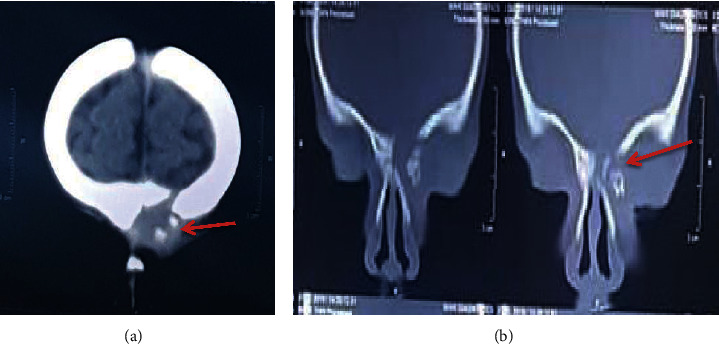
CT scan head of the infant. (a) Defect in the frontal bone; there is discontinuity of the left frontal bone with erosions. (b) Erosion of the frontal bone plate due to chronic postinfective etiology as seen on CT head, suggestive of PPT.
